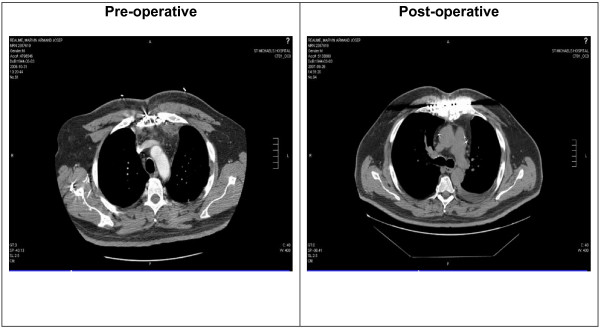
Pre and Post-operative chest CT scan following sternal plates' fixation. It demonstrates resolving of mediastinitis and sternal union.
